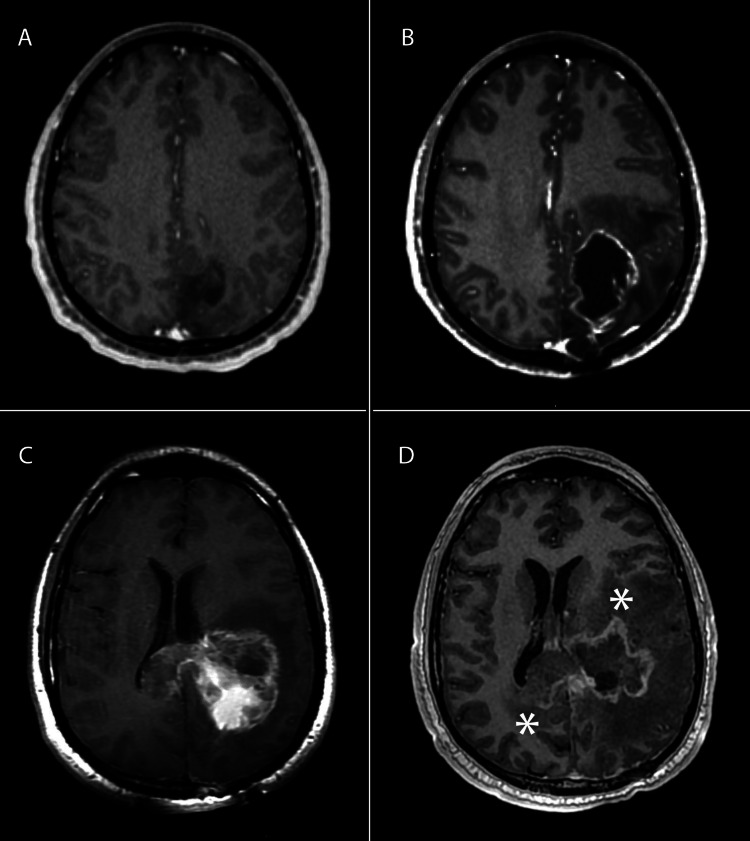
Radiographic evolution of patient’s disease. Contrast enhanced, axial T1 MRI images status post initial maximal safe resection of grade 3 astrocytoma four years prior to case presentation (A), status post second subtotal resection demonstrating GB (B), progression of secondary GB (C), status post maximal safe resection and GammaTile placement showing progression of anterior disease component and posterior internal capsule component as indicated by the asterisk (D).
